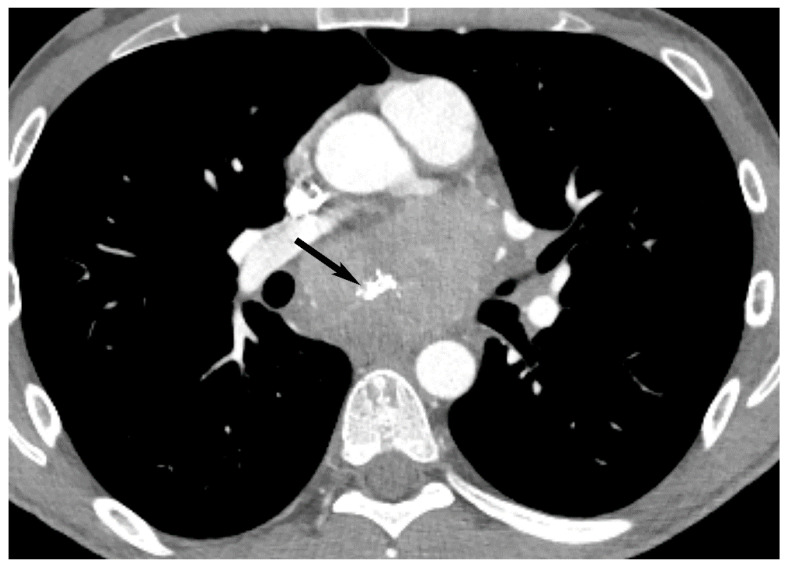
Castleman disease. CT shows hypervascular mass with a focus of calcification (arrow) in the visceral mediastinum. Biopsy showed Castleman disease.
